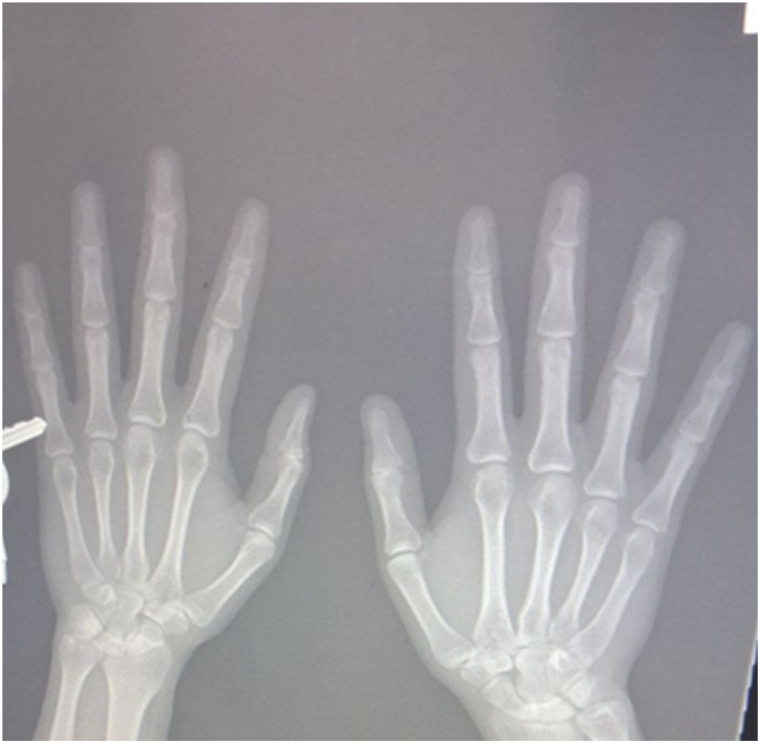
Normal hand radiograph showing no erosions, joint space narrowing, or bony abnormalities.
